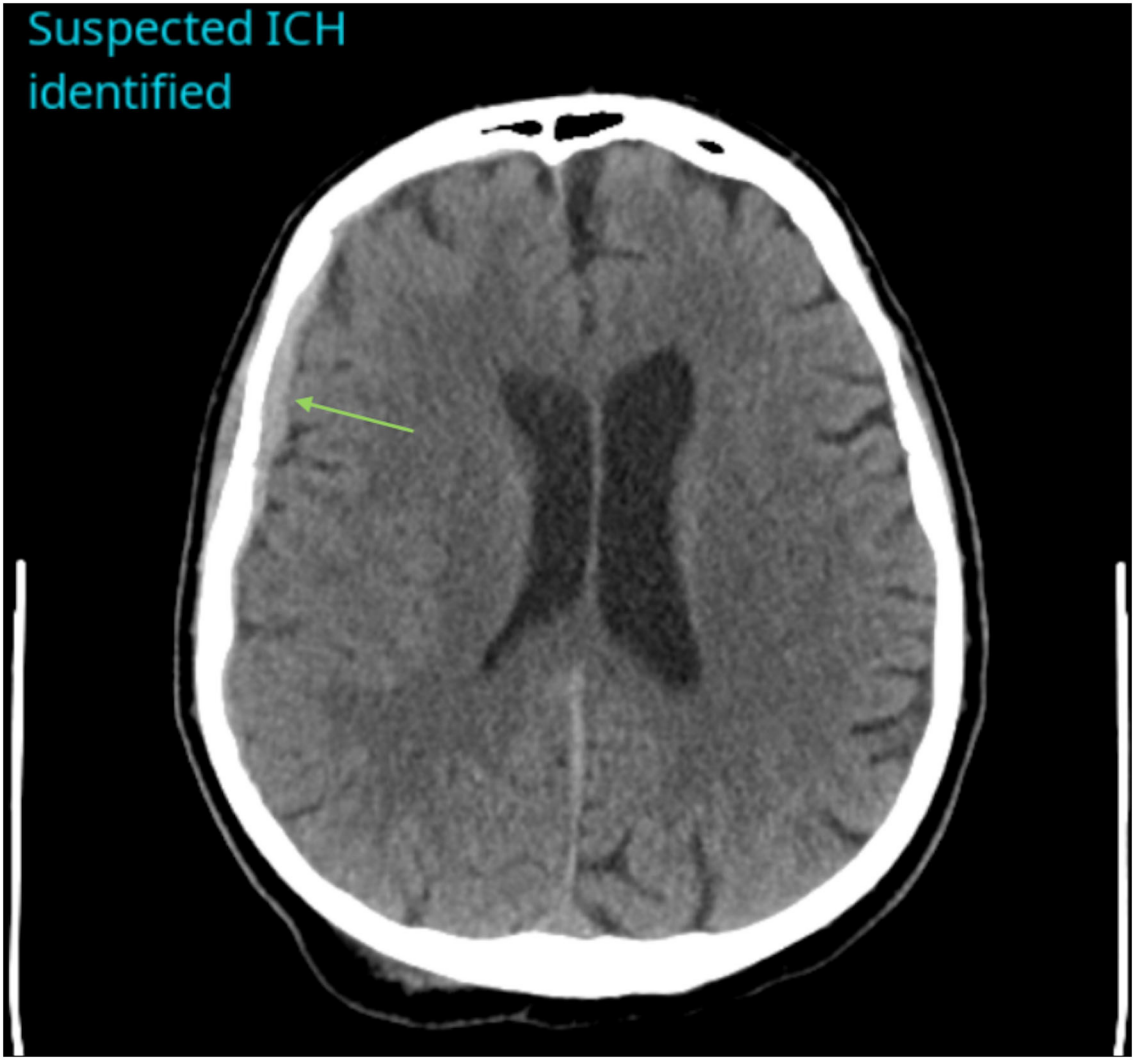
Example of a positive ICH identified by CINA®. There is an acute subdural hemorrhage along the right cerebral convexity on non-contrast CT (green arrow).
